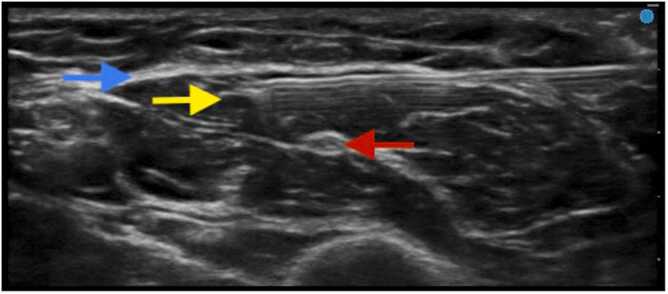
Ultrasound-guided deposition of local anesthetic deep to biceps brachii fascia (fascia labeled with blue arrow, implant with yellow arrow, and musculocutaneous nerve as red arrow). Depth markers are seen on the right side of the image (total depth of the ultrasound image is approximately 2.3 cm).
